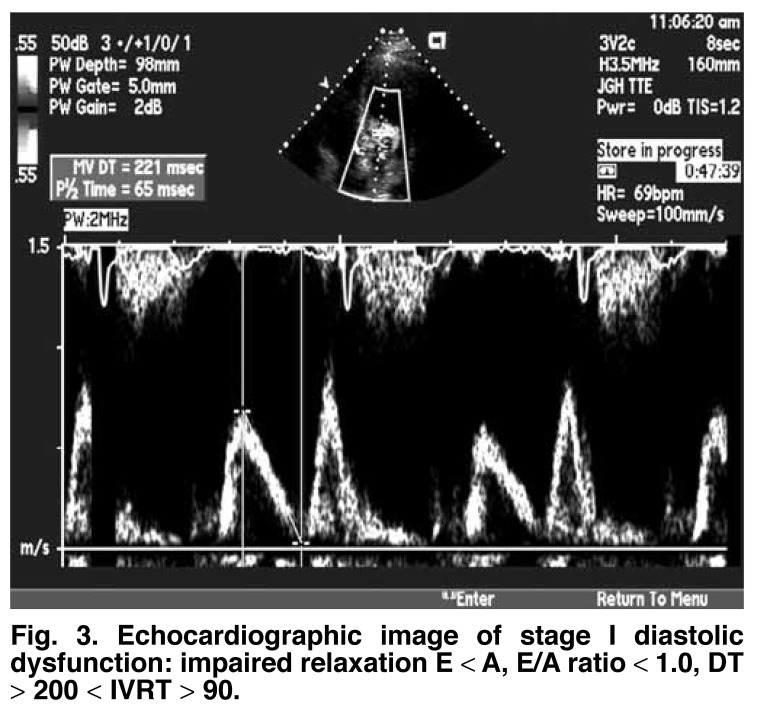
Echocardiographic image of stage I diastolic dysfunction: impaired relaxation E < A, E/A ratio < 1.0, DT > 200 < IVRT > 90.
